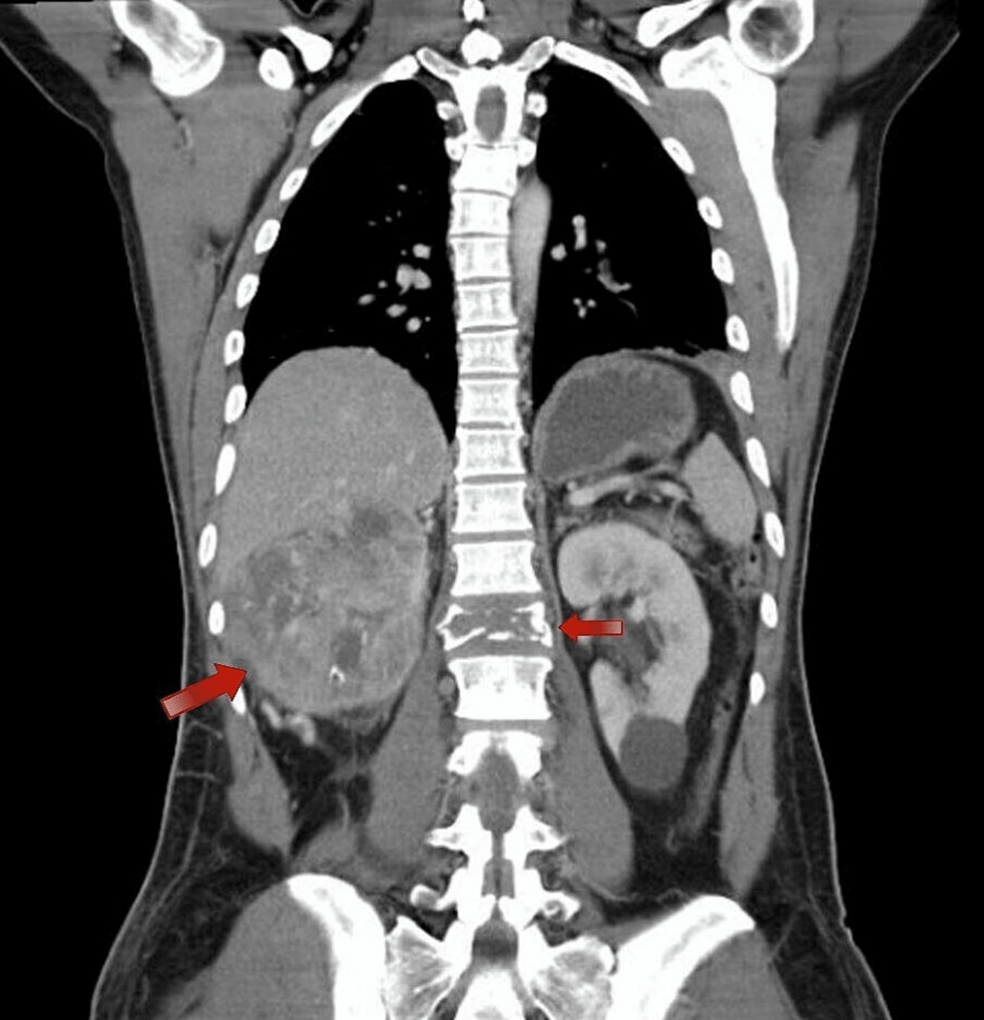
Coronal view of CT abdomen with IV contrast enhancement demonstrating right renal mass with concurrent L1 vertebrae fracture
